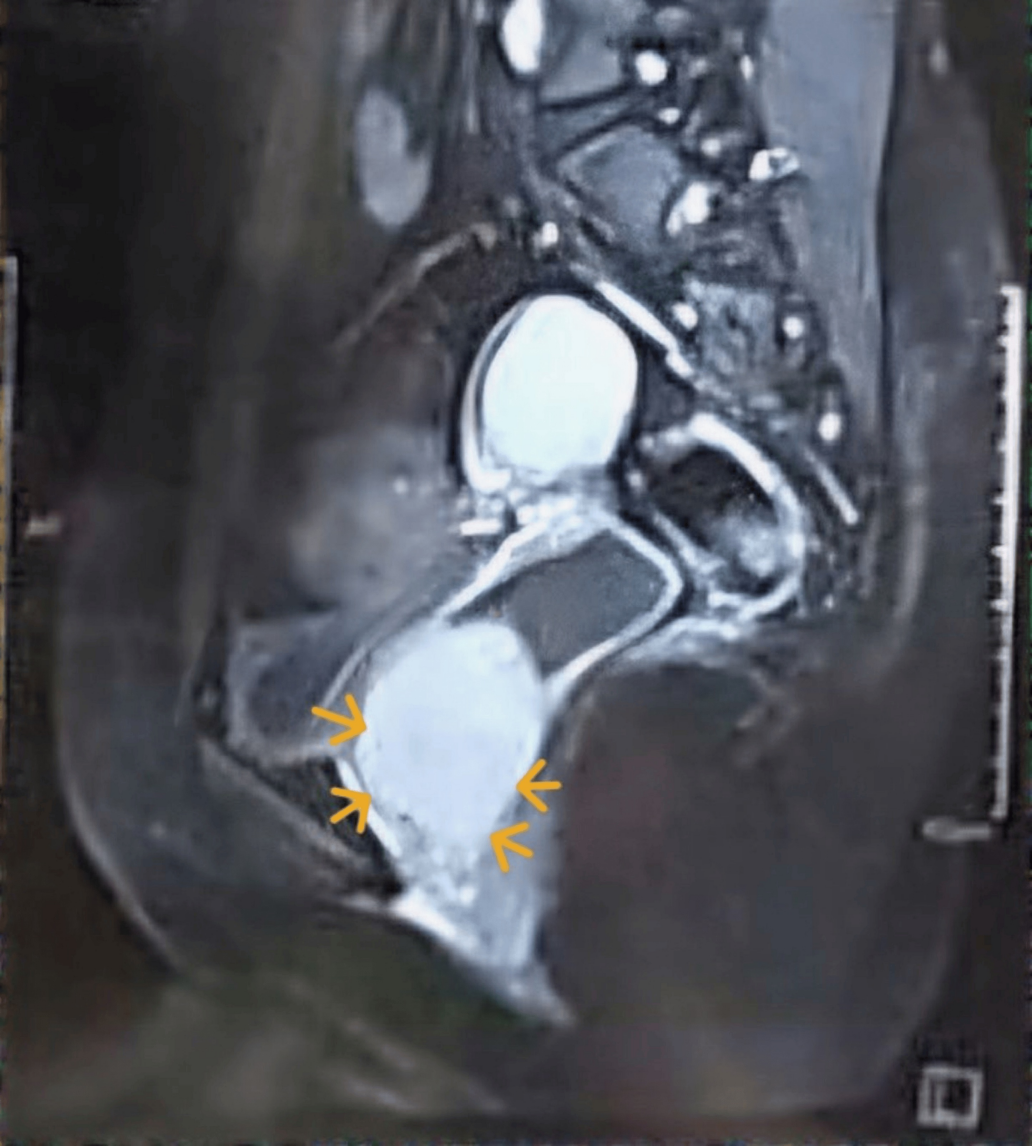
Magnetic resonance imaging with longitudinal view of the pelvis and perineum showing a hyperintense homogeneous mass arising from the anterior wall of vagina (marked by arrow)
